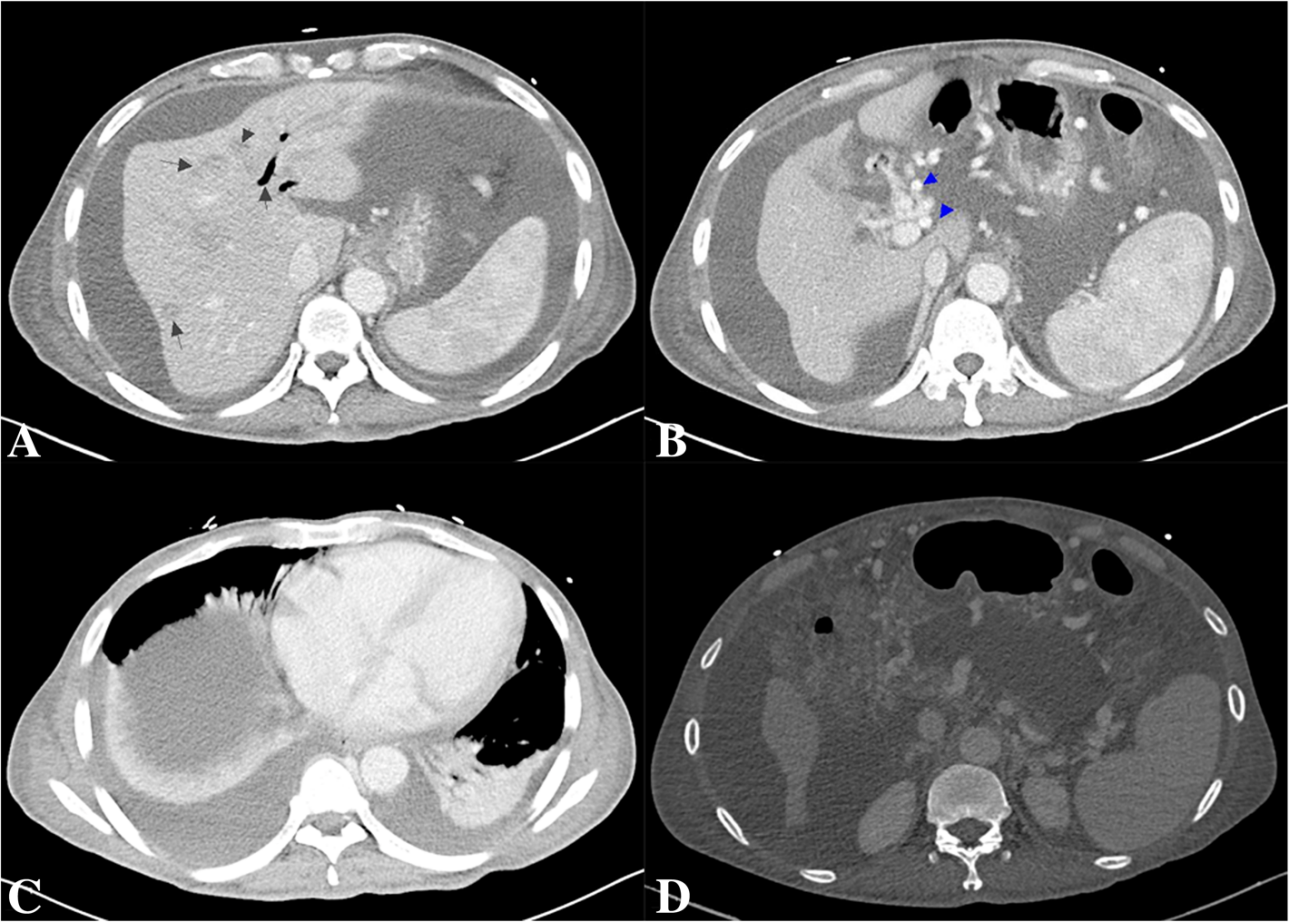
Abdominal computed tomography. Images of the liver showed numerous hypodense nodules with mild contrast enhancement, ascites and intrahepatic bile duct dilatation (a). There were multiple enlarged lymph nodes in the hepatoduodenal ligament around the abdominal aorta (b). Mesenteric lymph nodes with bilateral pleural effusion (c). Multiple nodular dense shadows with dilatation of the main pancreatic duct in the tail region (d)
